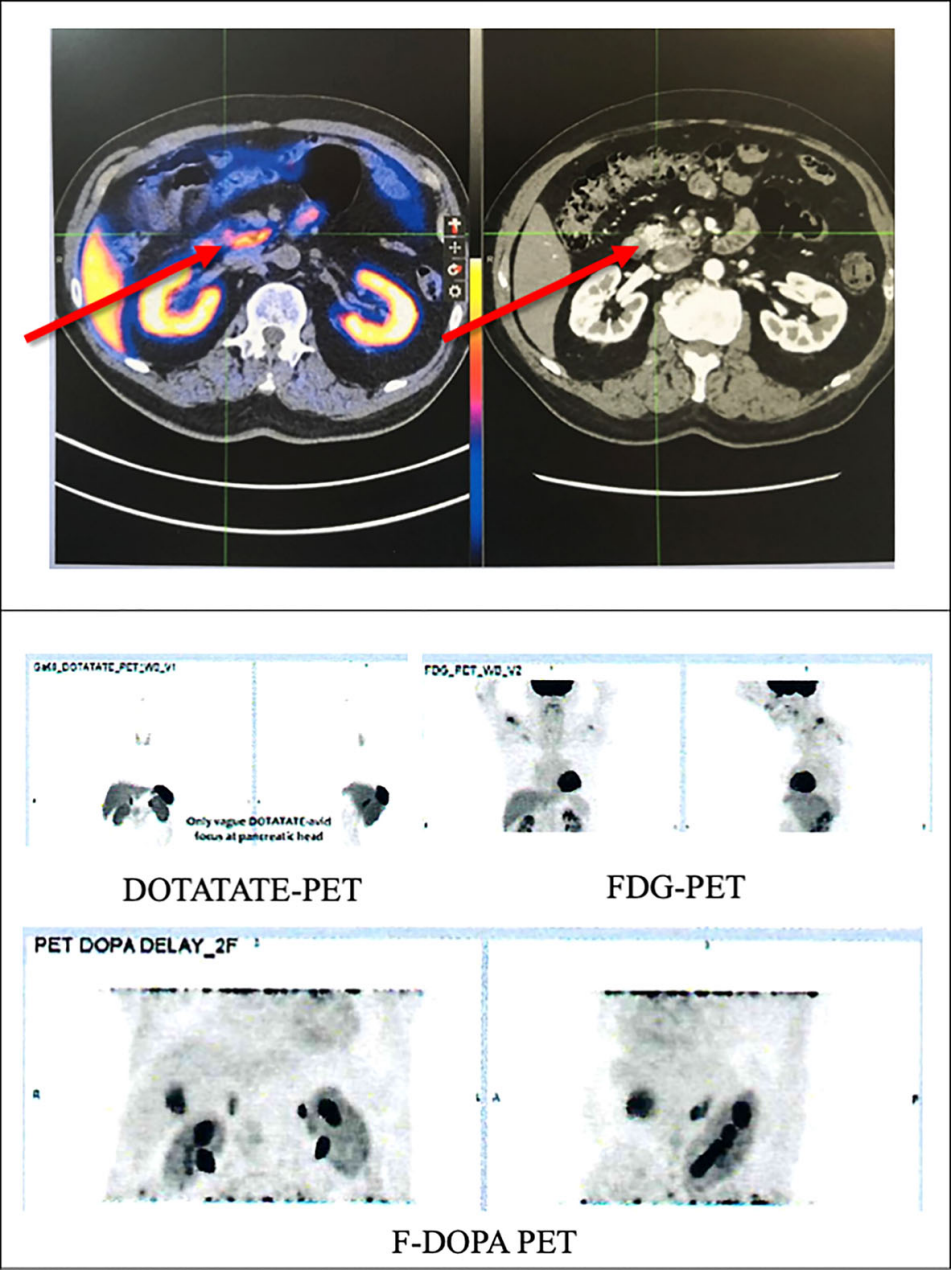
PETCT scan of the patient showing a tumour in the pancreatic head with vague DOTATATE avidity (red arrows) but without FDG or F-DOPA avidity. FDG, ^18^F-fluorodeoxyglucose; F-DOPA, ^18^F-fluorolevodopa (F-DOPA); PETCT, positron emission tomography-computed tomography.
